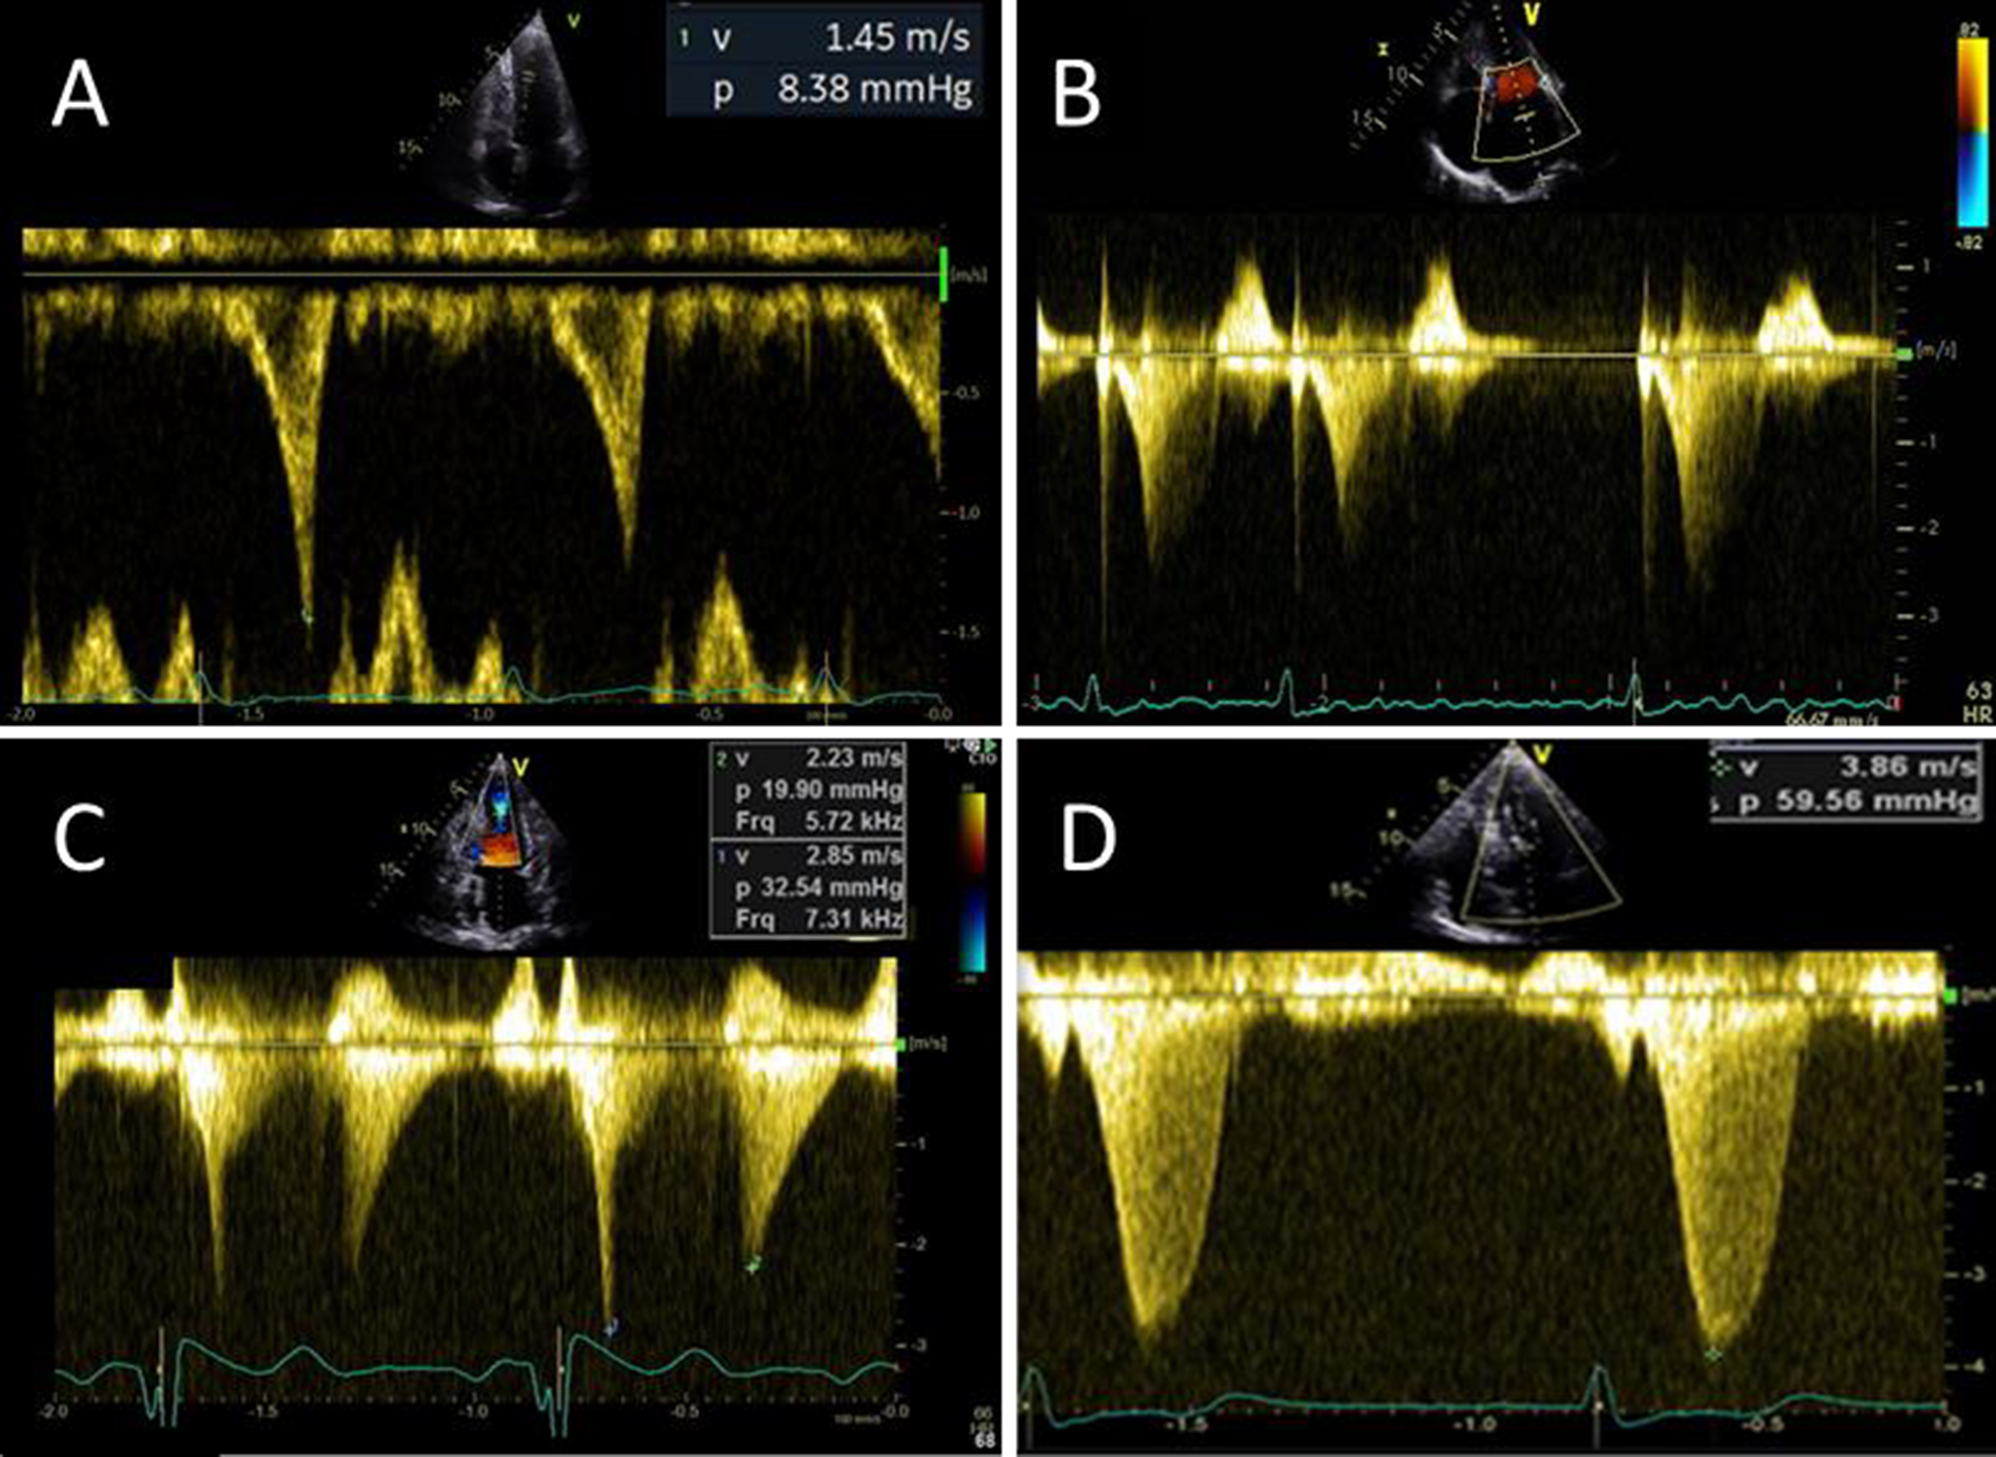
TTE images displaying four distinct Doppler profiles: Category 1 non-obstructive flow acceleration defined as either early or mid-systolic peak without being holo-systolic (A); Category 2 early-obstructive prolonged apical emptying defined as persisting throughout systole and <30 mmHg (B); Category 3 overt obstructive Doppler signal void with paradoxical jet defined as two distinct jets in systole and paradoxical diastolic jet of any gradient (C); and Category 4 high velocity complete spectral jet defined as holo-systolic and >30 mmHg (D). TTE, transthoracic echocardiography; LVMCO, left ventricular mid-cavity obstruction
